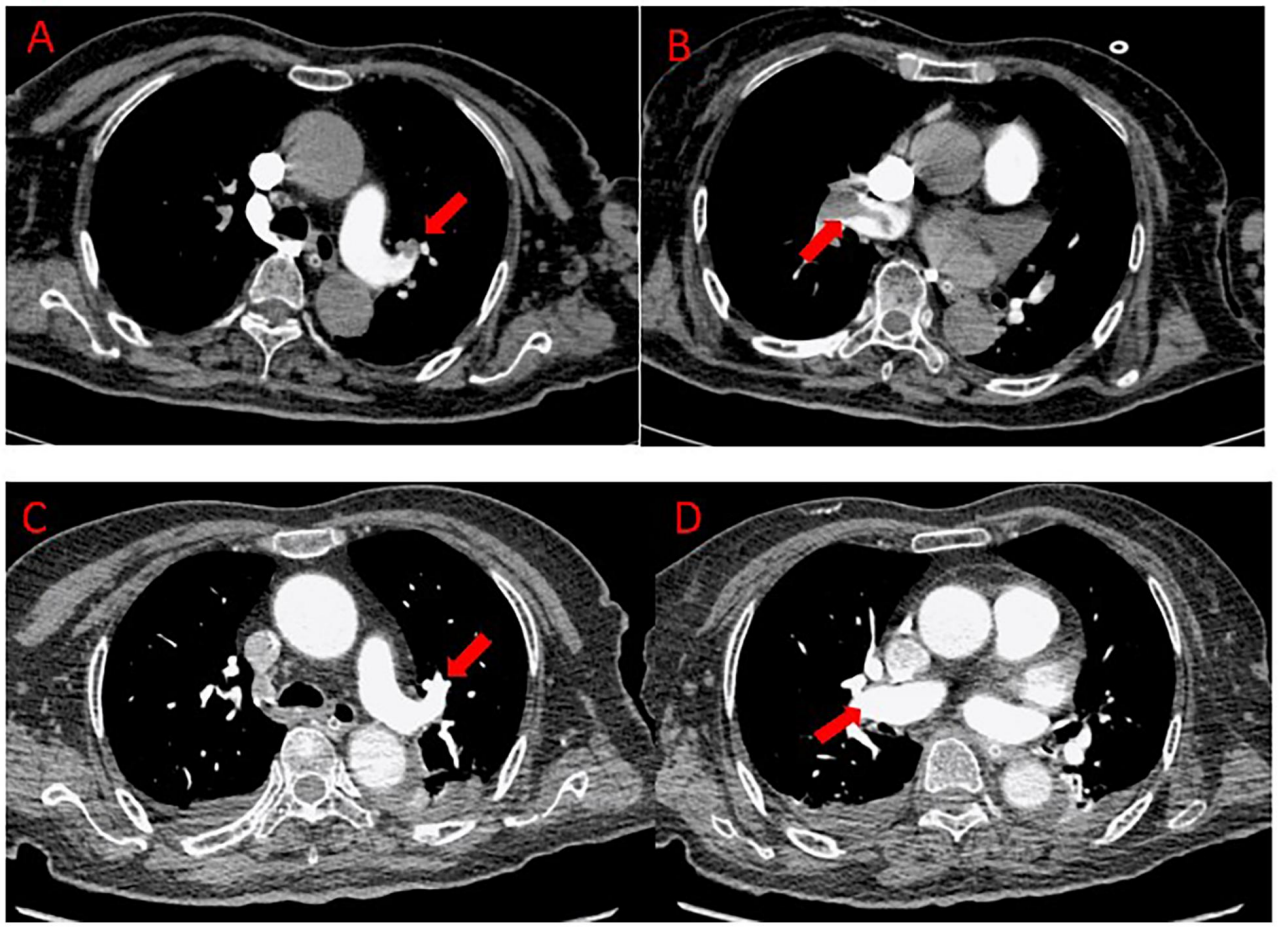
CT pulmonary angiography images. (A,B) Axial CTPA images on day 17 after cerebral thrombectomy (at presentation of PE): large filling defects (arrows) are seen occluding the right main pulmonary artery and its branches, diagnostic of acute massive PE. (C,D) Follow-up axial CTPA images on day 7 after pulmonary aspiration thrombectomy: near-complete resolution of the previously seen filling defects, indicating successful mechanical thrombus removal and restoration of pulmonary arterial flow.
